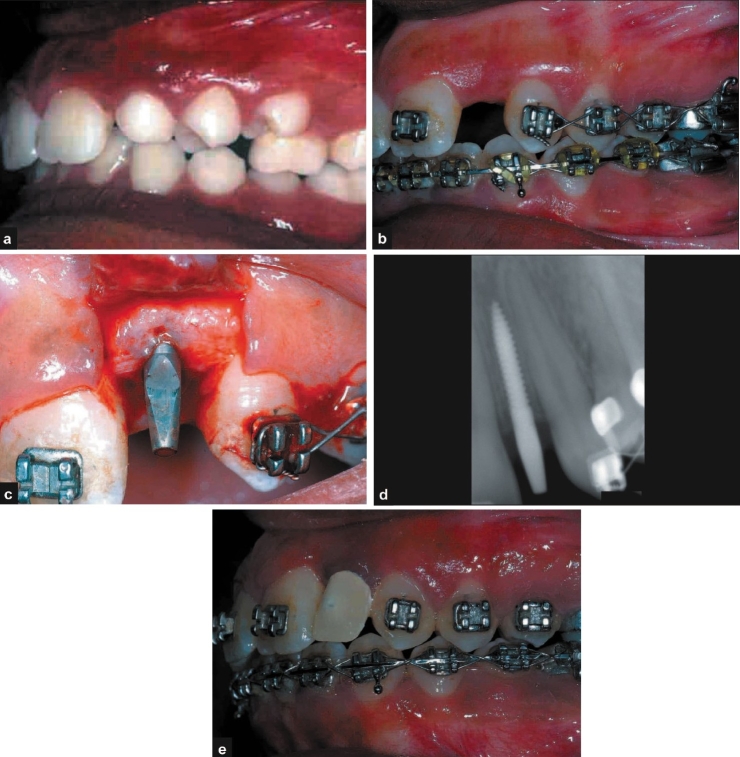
a, b) Pre-operative photographs; c, d) operative photograph and radiograph; e) post-operative photograph
